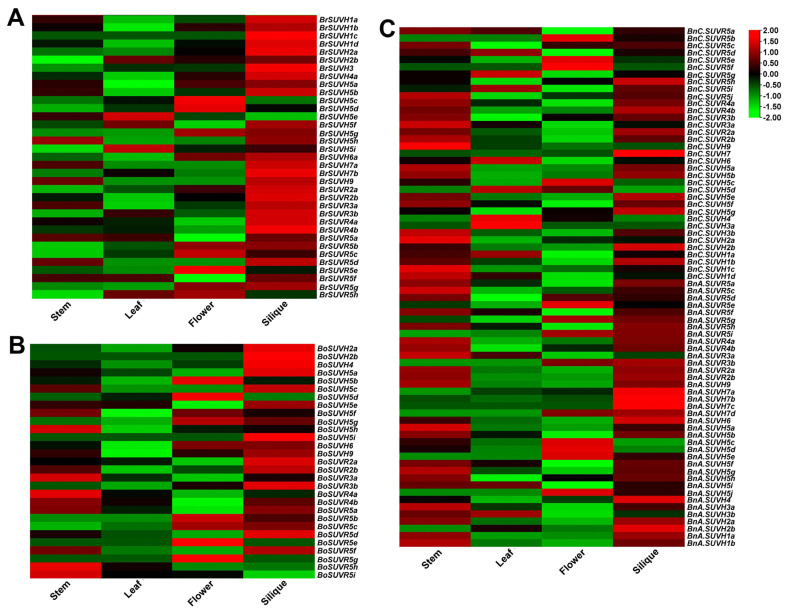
Expression patterns of SUV genes in four tissues (stem, leaf, flower, silique) of *B.*
